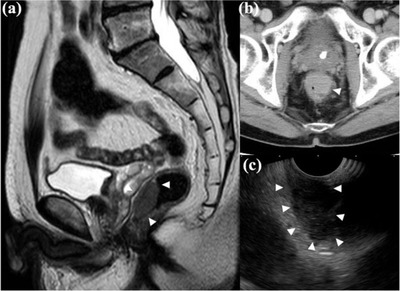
(a) T2‐weighed magnetic resonance imaging showing a well‐defined, elevated lesion in the anterior rectal wall. (b) Contrast‐enhanced computed tomography shows a lower rectal mass with a contrast‐enhancing effect. (c) Endoscopic ultrasonography demonstrates a heterogeneous hypoechoic lesion.
